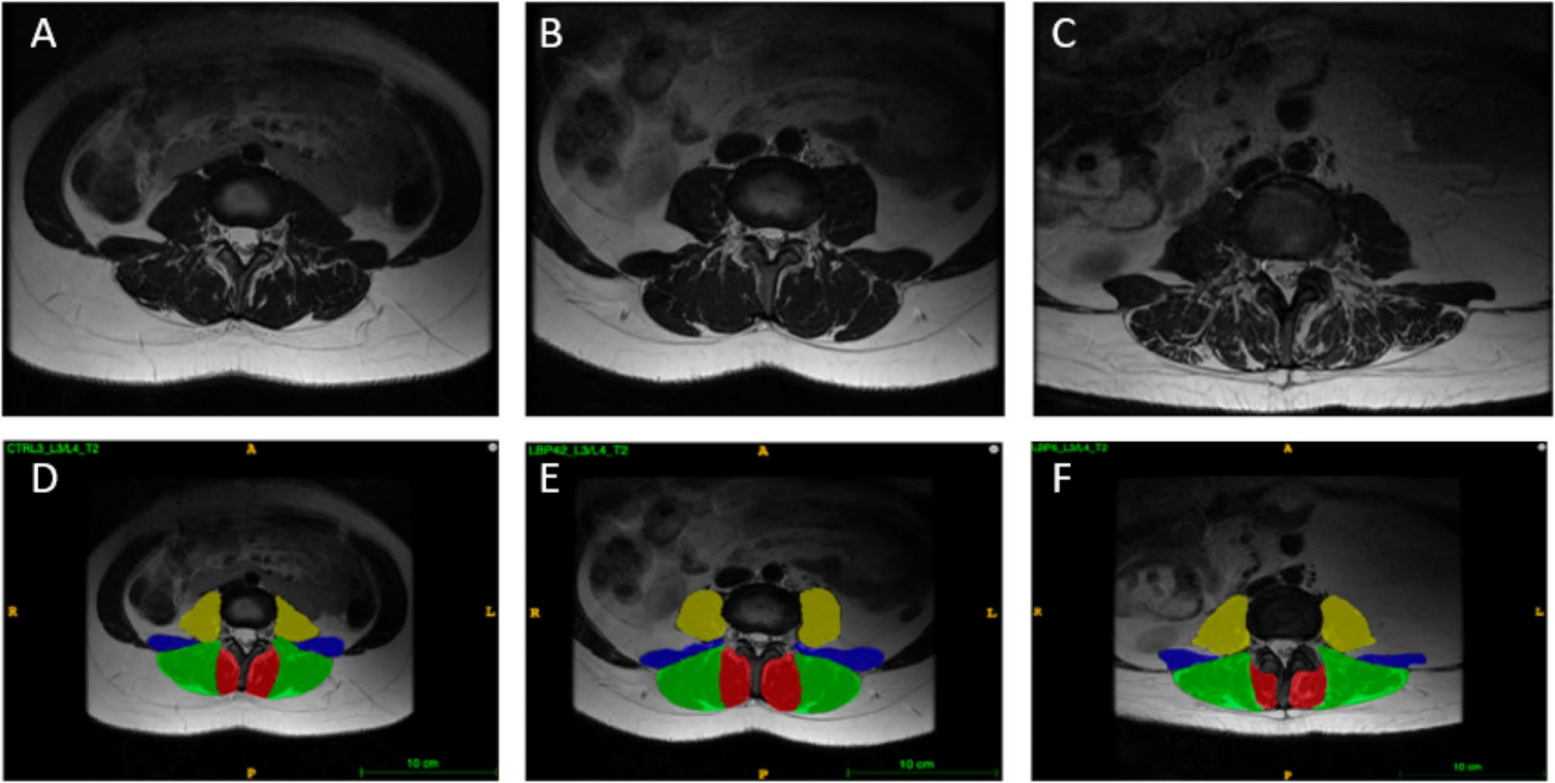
All images are T2-weighted images at the L3/L4 level in females. The first column (A & D) are images of the control. The middle column (B & E) are images of an age-matched individual with LBP. The third column (C & F) are images of the older individual with LBP. D, E, and F include the segmentations of the lumbar multifidus (red), erector spinae (green), quadratus lumborum (blue), and psoas (yellow) muscles
